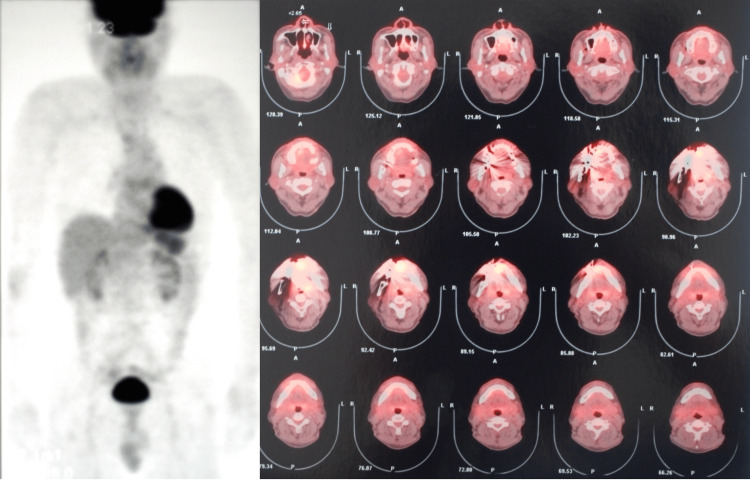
FDG-PET/CT scans performed after the postoperative definitive diagnosis showing no cervical lymph node or distant metastases FDG - fluorodeoxyglucose
